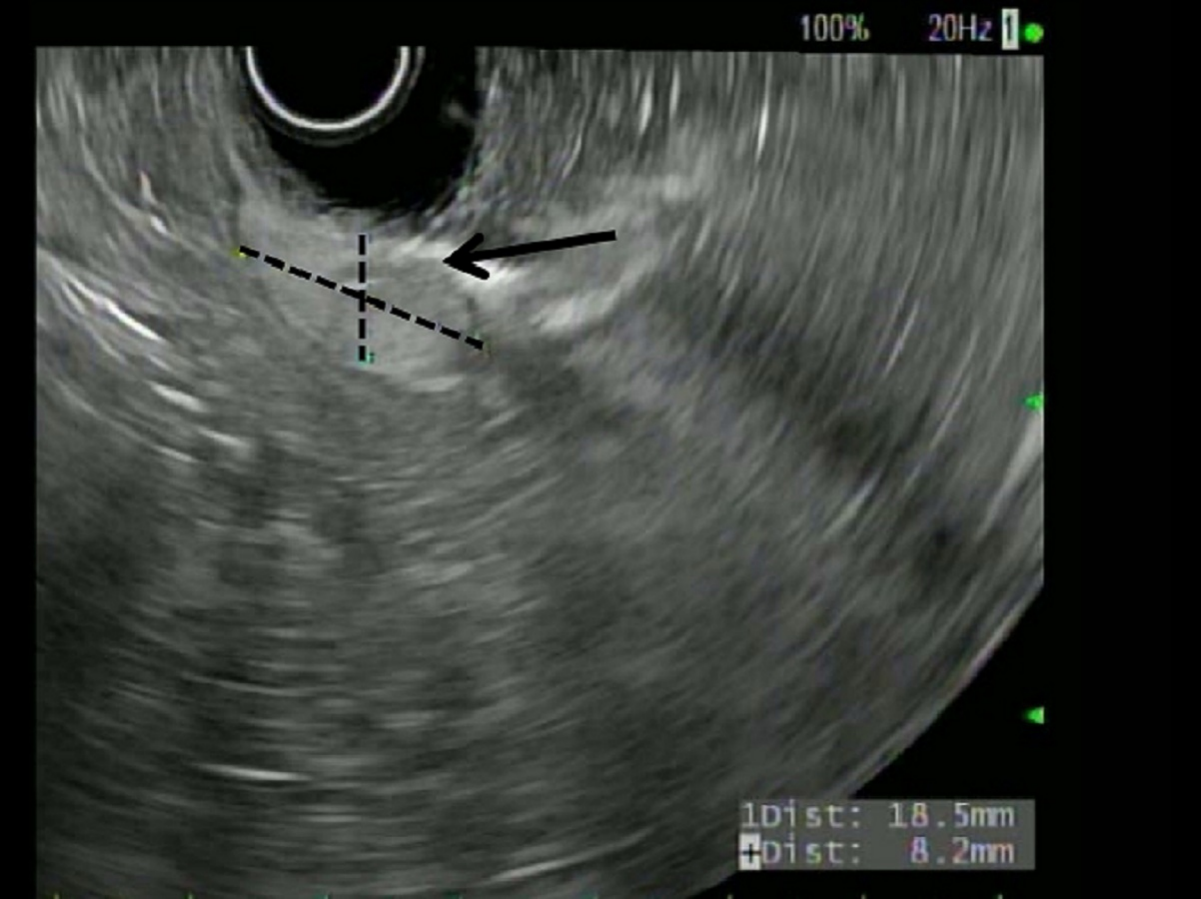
Endoscopic ultrasound showing a reduction in size of the lipoma after argon plasma coagulation (dotted lines)
